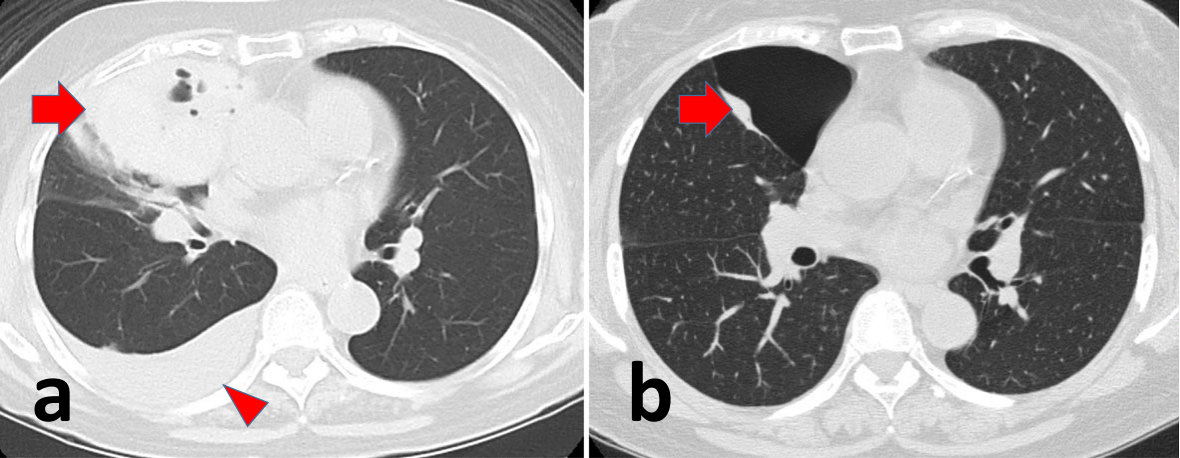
a A chest CT on admission revealed an air-fluid mass (arrow) adjacent to the right upper and middle lobe with a pleural effusion (arrowhead). b A CT scan obtained 15 years prior revealed a cyst(arrow)at the corresponding site
